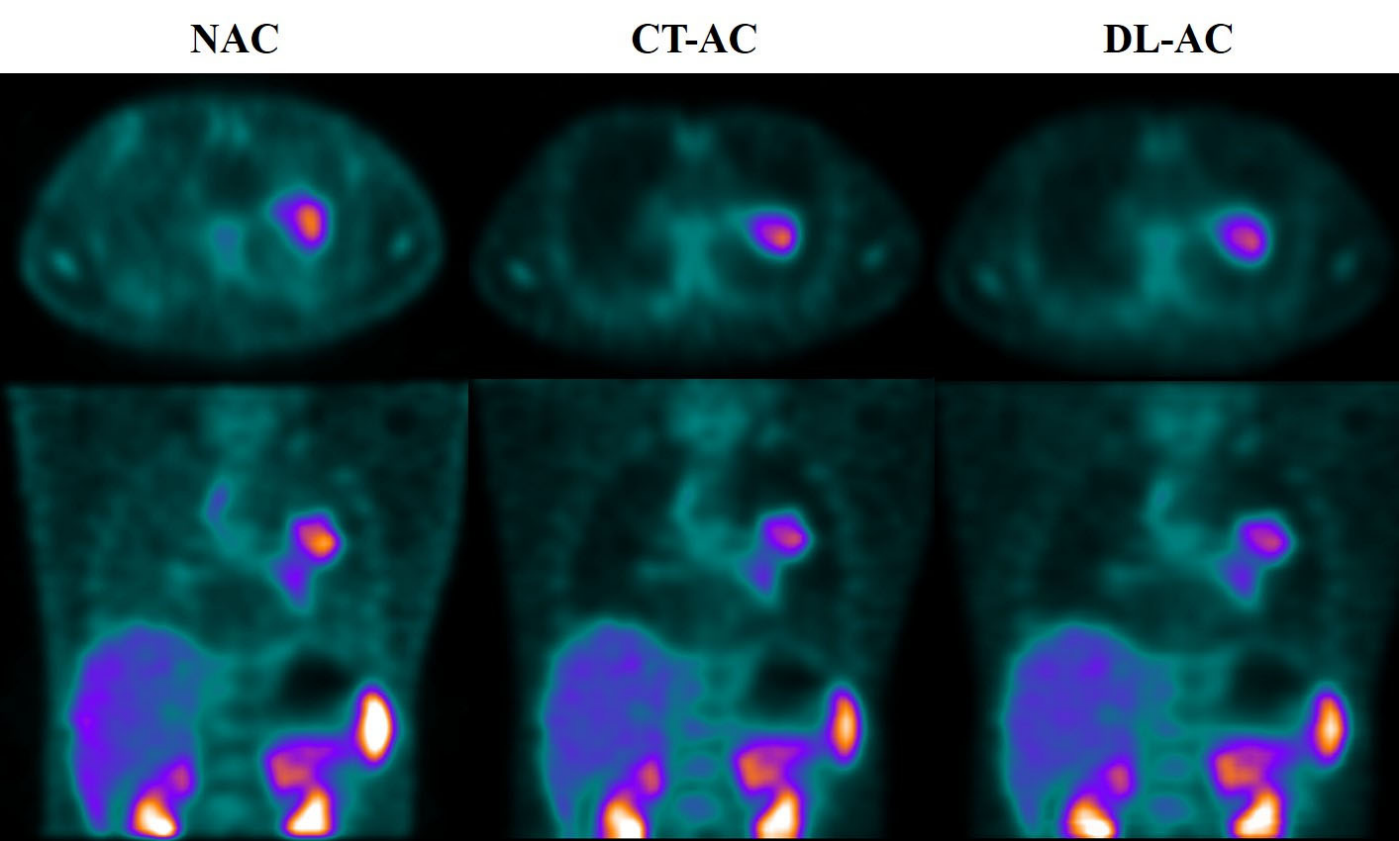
The error image between CT-AC and DL-AC in the SPECT axial image of another patient. The green color indicates that the error is 0, and the red color indicates that the error is maximum.
